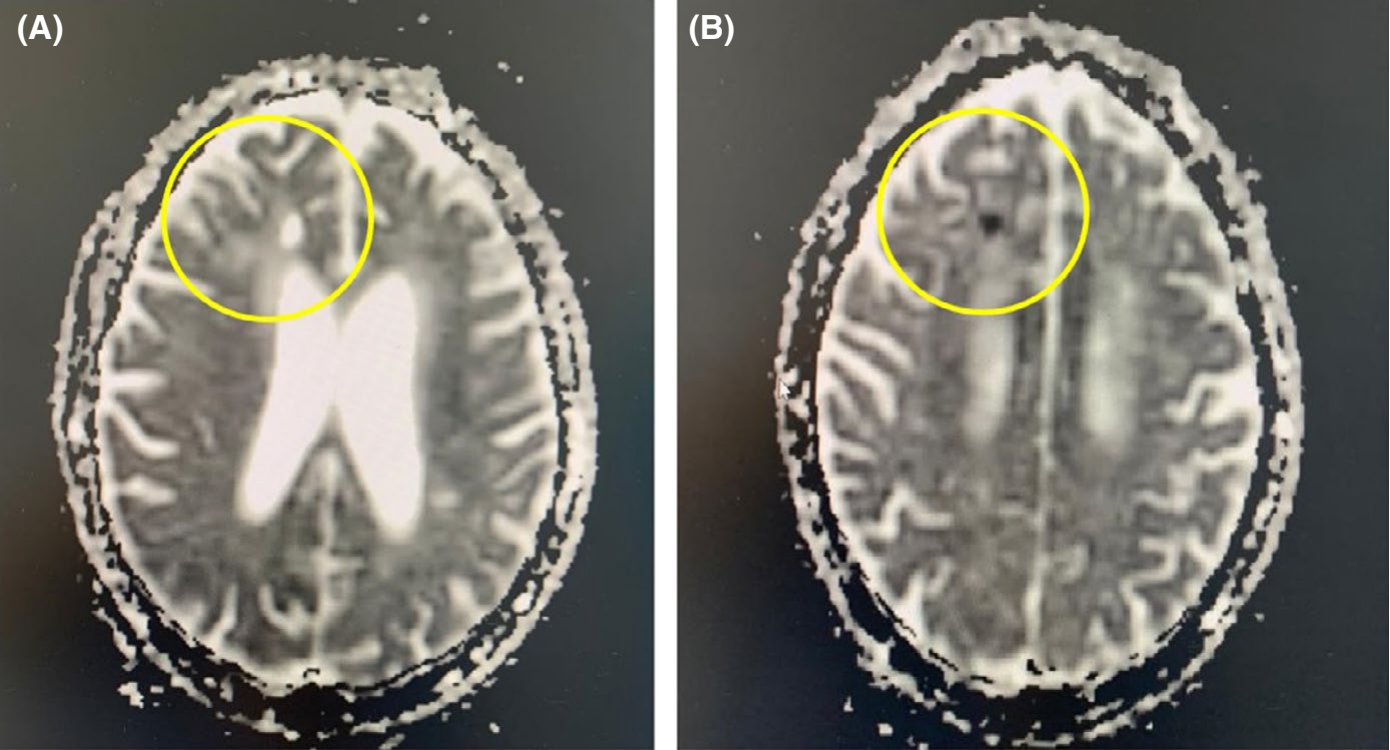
Brain MRI showing an hypersignal diffusion in the right frontal lobe (A) with a decrease of the apparent diffusion coefficient (ADC) in favor of a recent ischemic stroke (B)
